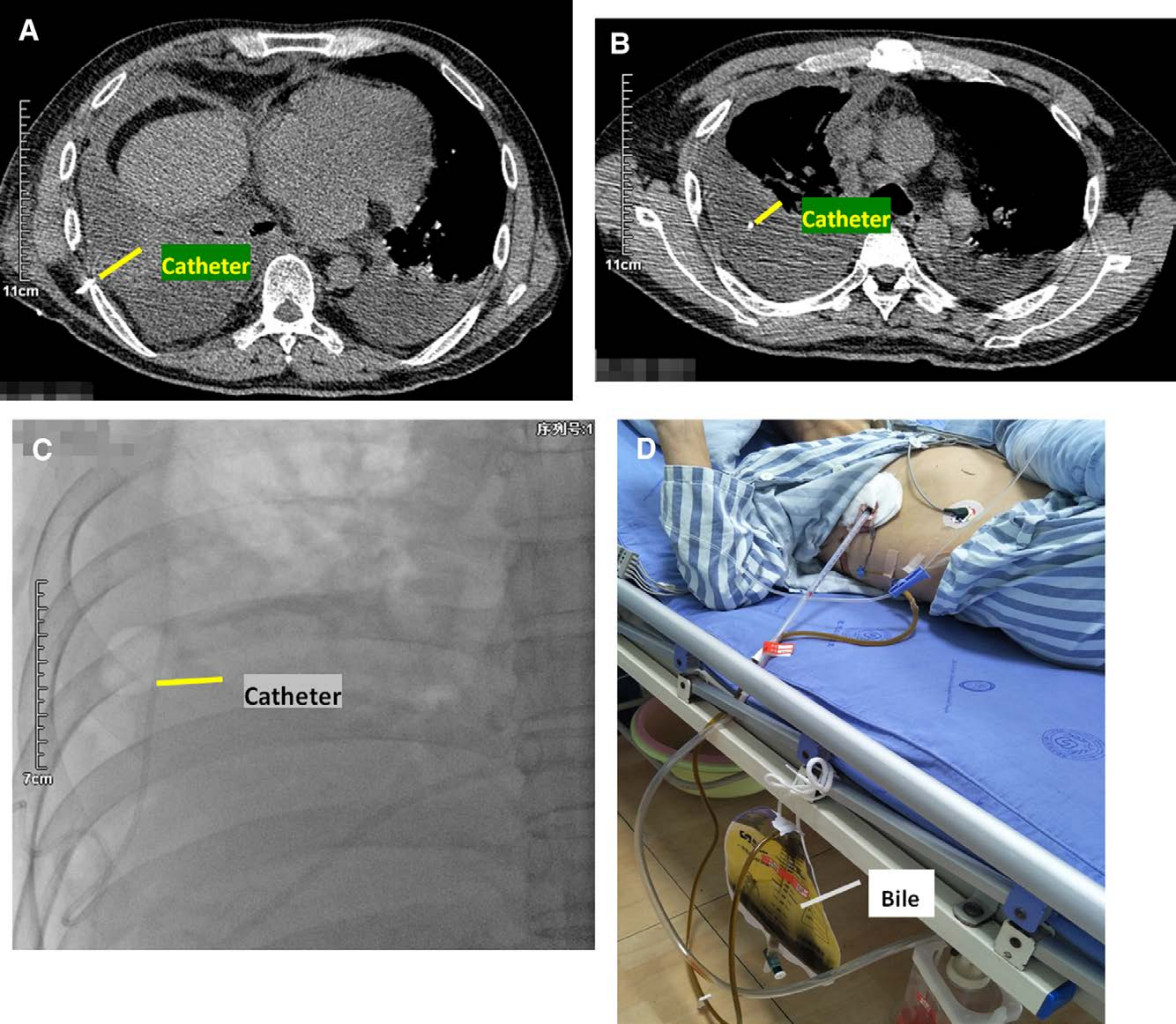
(A and B) Chest computed tomography showing a right pleural effusion and the pleural puncture catheter (as indicated by the line). (C) X-ray shows that the tip of the catheter is facing the head side, which can exclude the bile duct from entering the chest through the diaphragm. (D) A large amount of bile was drained from the catheter in the patient’s chest cavity.
